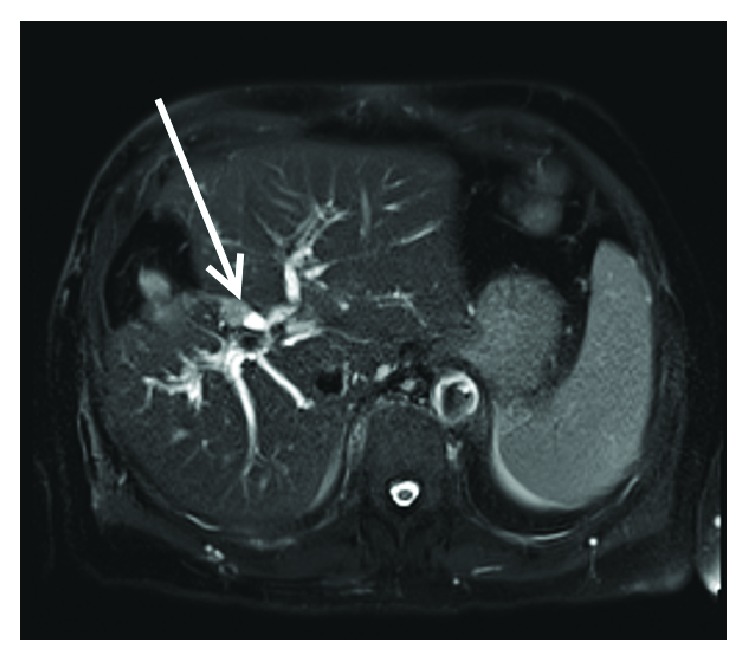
MRI showing the intrahepatic lesion.
